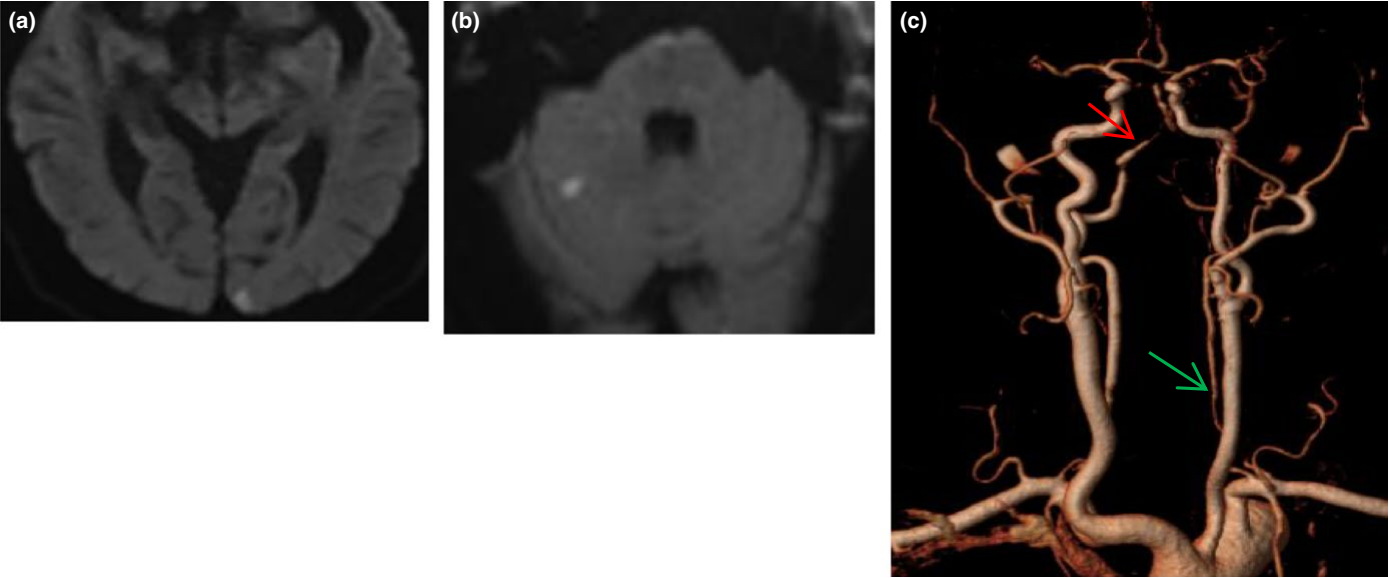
A 70‐year old man with acute vertigo and vomiting arrived ED 2.5 hr after onset and received MRI 28h after onset; his NIHSS was 0 during this time. Cerebral MRI showed left occipital (a) and right cerebellar hemisphere(b) infarction, left vertebral artery was narrow (green arrow) with right vertebral artery stenosis (red arrow, c)
